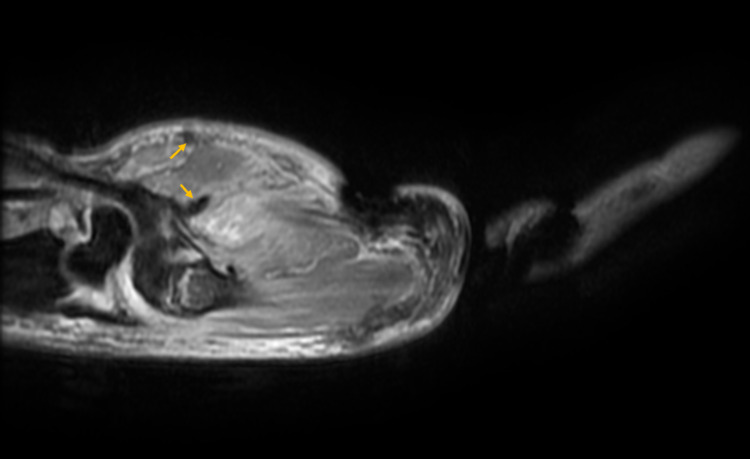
MRI showing small, non-enhancing lesions (arrows) in the thenar musculature and edema in the metacarpals and wrist.
